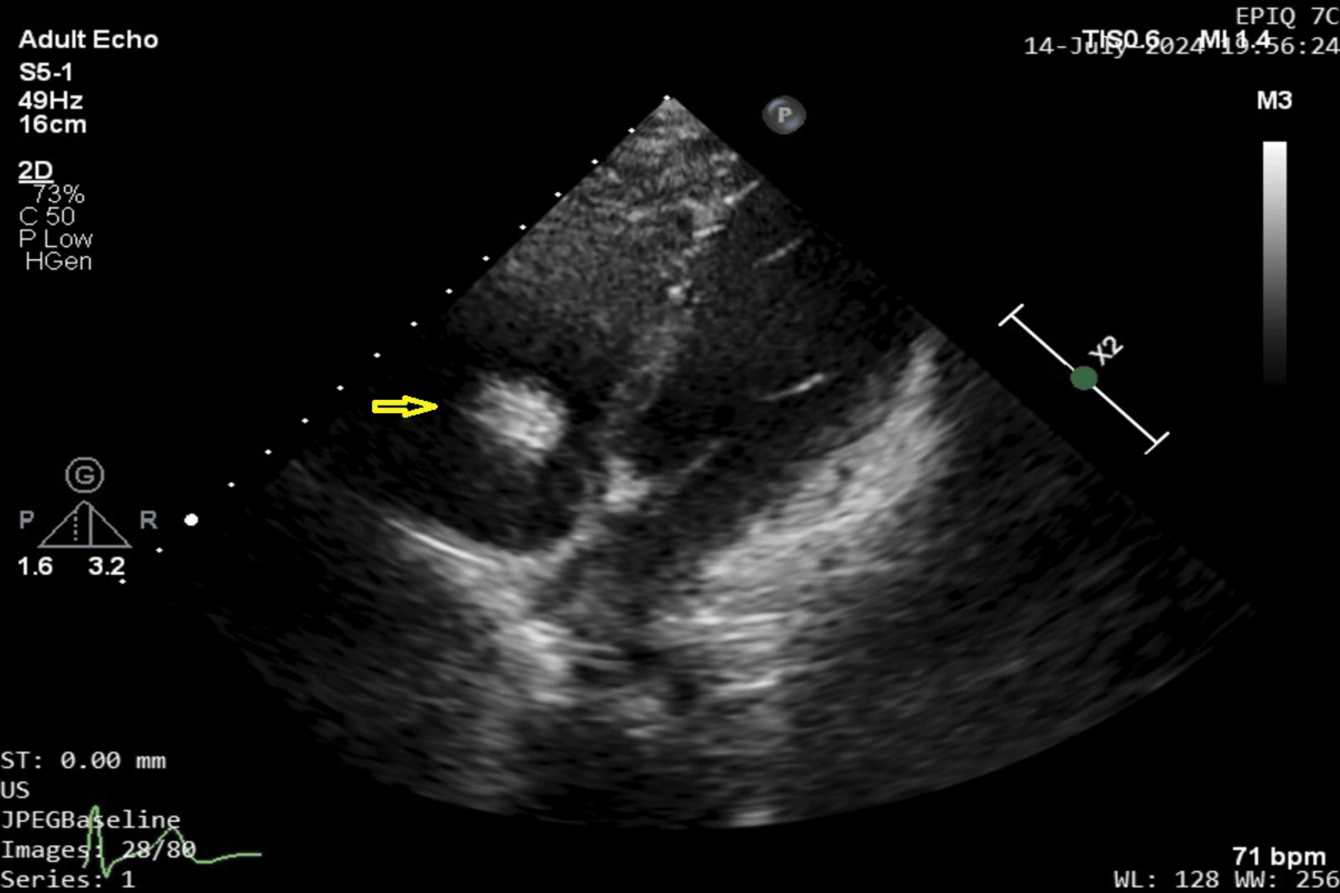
Modified 4-chamber view on echocardiography suggestive of a hyperechoic mass (yellow arrow) measuring 4 x 6 mm attached to right atrial aspect of tricuspid leaflet.
